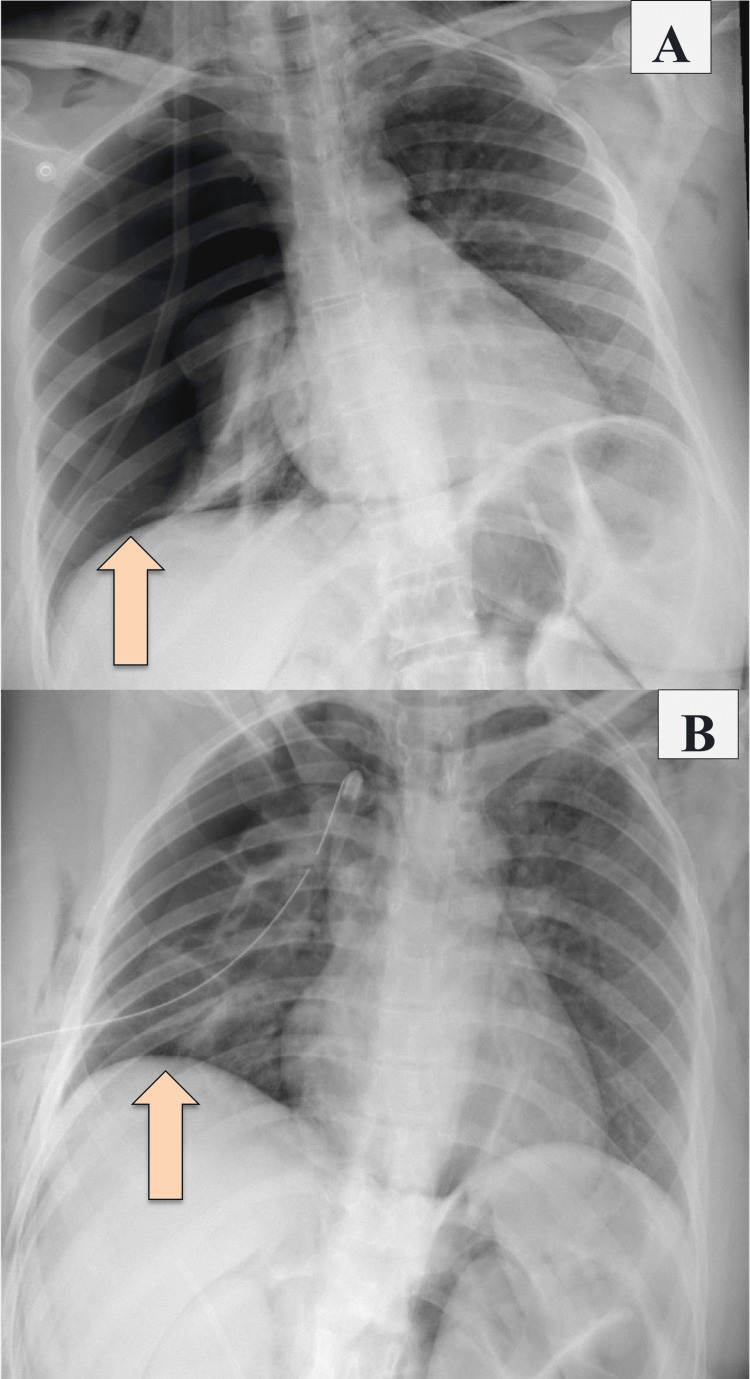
Chest X-ray showing right pneumothorax and pneumediastinum pre- and post-chest tube insertion A: Before chest tube insertion (the arrow is pointing at the pneumothorax before tube insertion); B: After chest tube insertion (the arrow is pointing at the resolution of pneumothorax after tube insertion)
